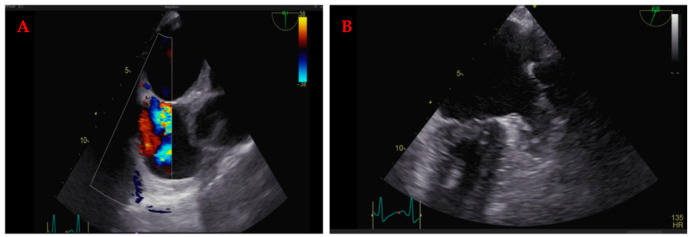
(A,B) Transesophageal ultrasound. (A) Biconcave section—small hole visible in the “primum” portion of the interatrial septum, connected to the membrane in the left atrium. (B) Transesophageal ultrasound-recent thrombosis at the level of the LAA.
